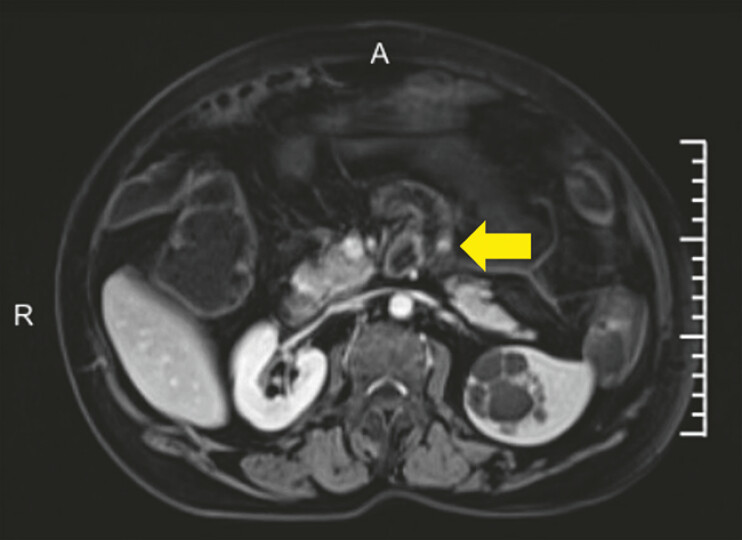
MRI showing abnormal signal nodules in the proximal jejunum.
